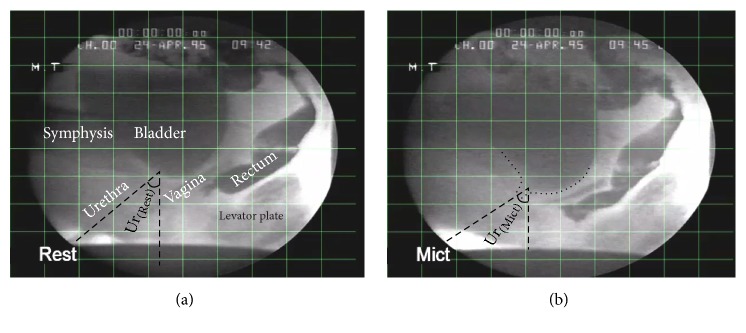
X-ray examination of an asymptomatic female pelvic floor urethral angle (a) Ur_(Rest)_ at rest and (b) Ur_(Mict)_ during micturition [77].
